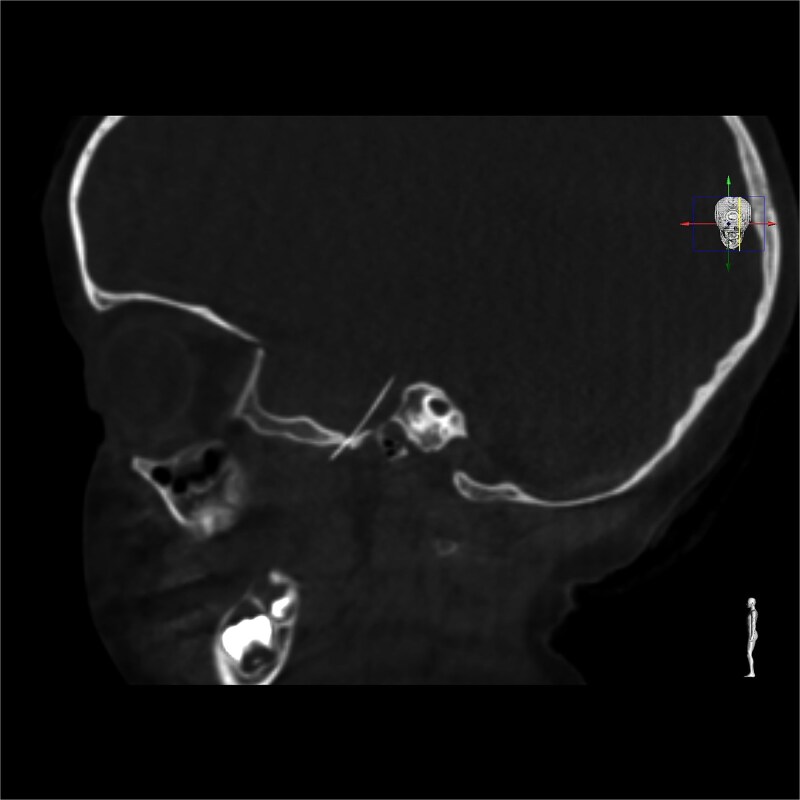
Intracranial CT scan showing the intracranial migration of the needle through the foramen ovale.
